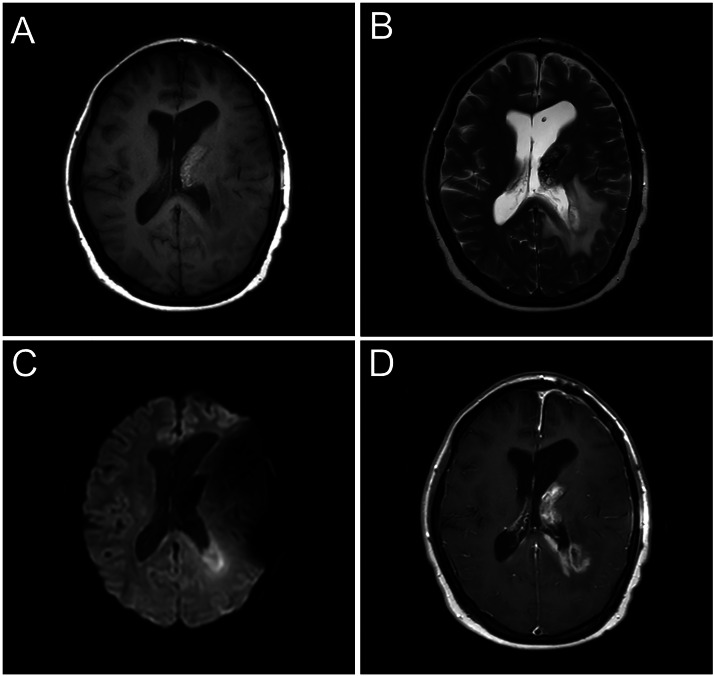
Brain MRI 14 months after initial presentation. The residual tumor in the left lateral ventricle appears hyperintense on T1-weighted imaging (A) and hypointense on T2-weighted imaging (B) relative to white matter. Diffusion-weighted imaging (b = 1000 s/mm²) (C) shows a newly emerged area of high signal around the trigone of the left lateral ventricle. Postcontrast T1-weighted imaging and (D) reveals contrast enhancement in both the residual lesion and the peritrigonal region of the left lateral ventricle.
